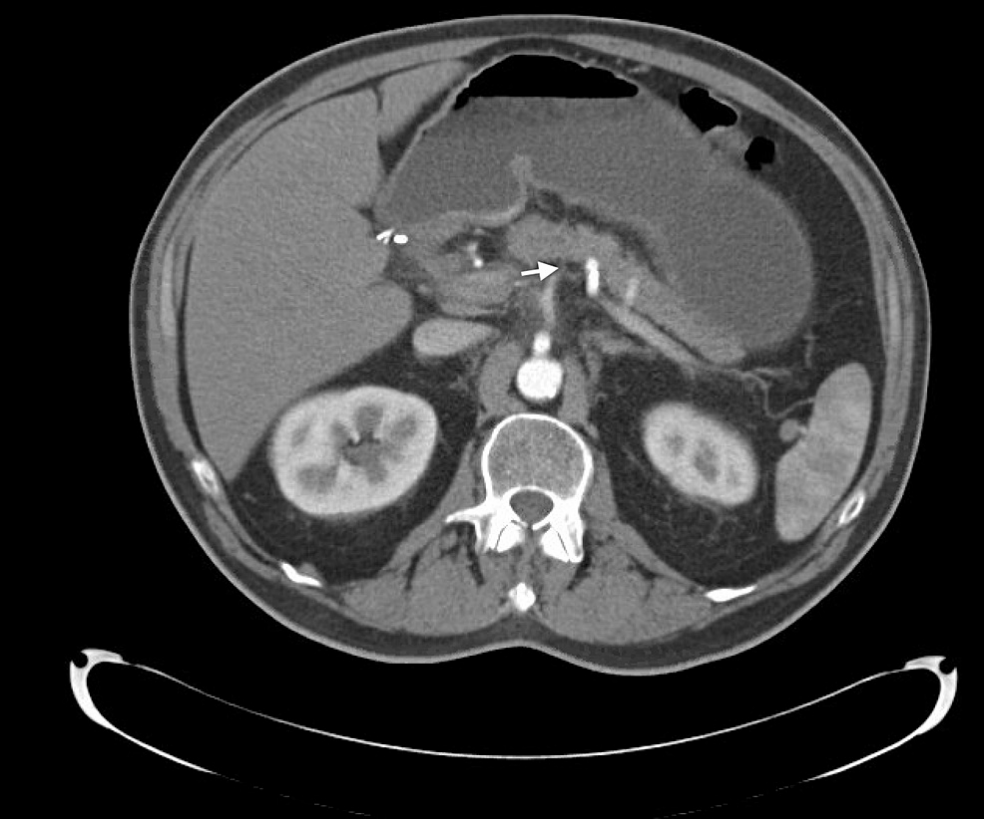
CT of the abdomen: interval resolution of previously seen fat stranding surrounding the pancreas (white arrow).
